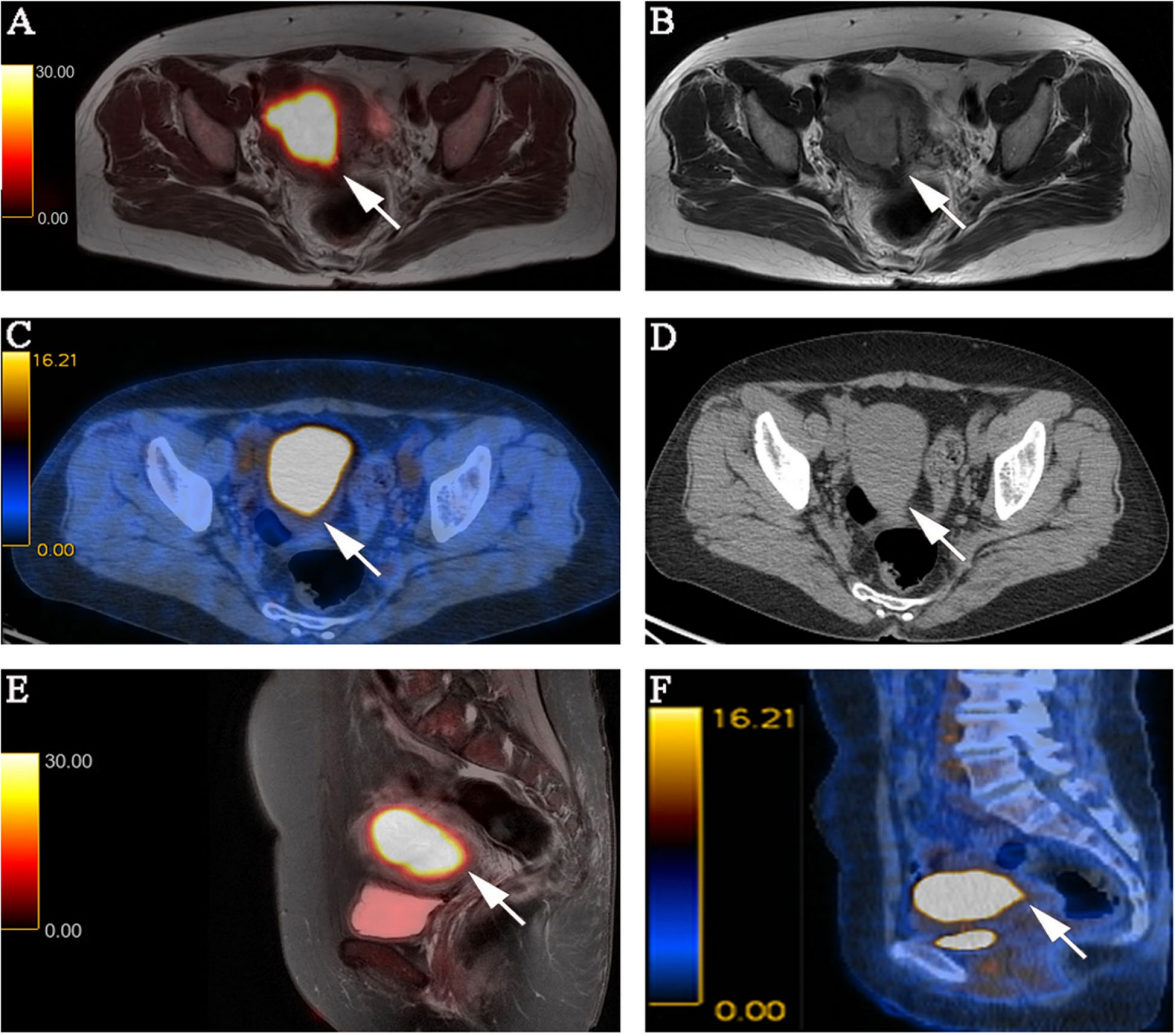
FIGO stage II pathologically confirmed moderately differentiated adenocarcinoma in a 57-year-old female patient. A Axial PET/MR images. B Axial MR T2-weighted images. C Axial PET/CT images. D Axial CT images. E Sagittal PET/MR images. F Sagittal PET/CT images. PET/MRI and MRI show the endometrial carcinoma invading the cervical stroma. PET/CT also shows the endometrial carcinoma invading the cervical stroma, while CT is difficult to determine the extension of the tumor. The PET/MRI and PET/CT staging were consistent with the pathological stage, namely FIGO stage II. The white arrow indicates endometrial carcinoma. FIGO, International Federation of Gynecology and Obstetrics; PET, positron emission tomography; MRI, magnetic resonance imaging; CT, computed tomography
